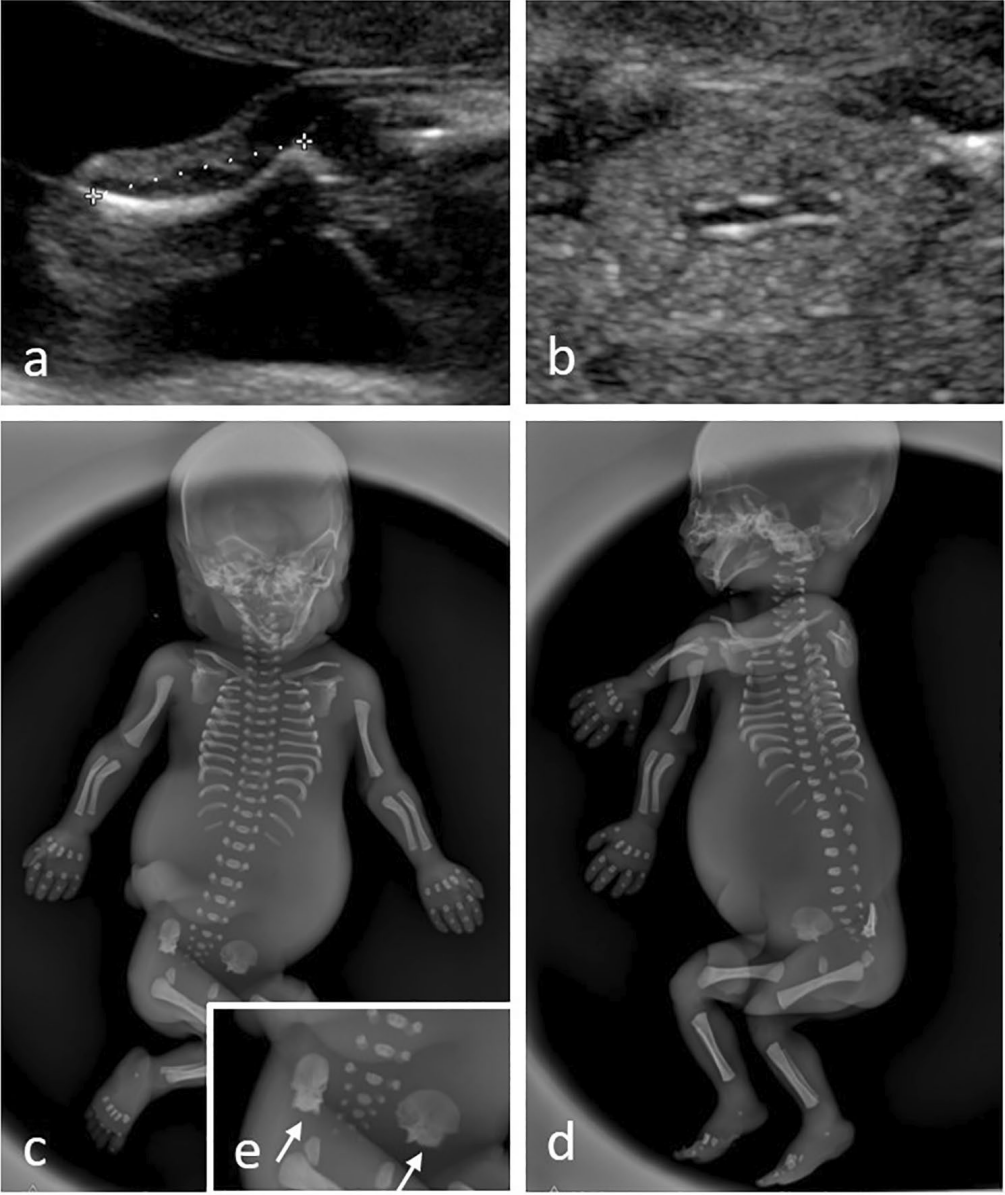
Pre- and postnatal (post-mortem) imaging in a fetus with Jeune syndrome: prenatal ultrasound showed a micromelia with a curved femur as well as b kidneys with missing corticomedullary differentiation at 21 + 3 weeks; c–e postnatal (post-mortem) radiography of the stillborn (TOP at 22 + 3 weeks): note the micromelia with the curved long bones as well as short ribs; e shows the typical “trident” appearance of the acetabular roof (white arrows)
